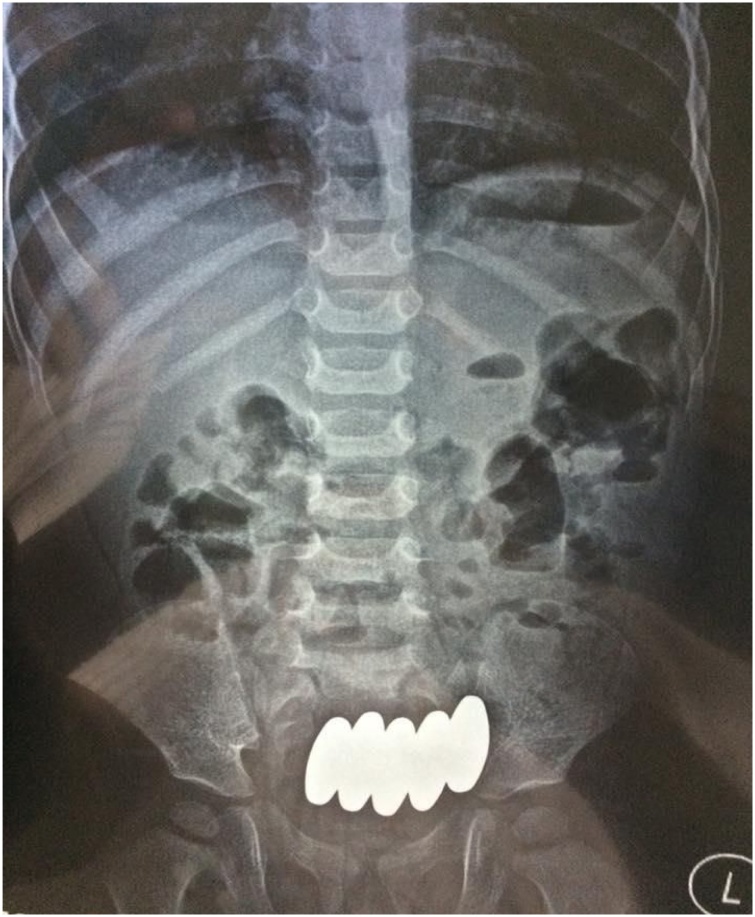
An erect abdominal X-ray showing five bullet shaped magnets in the lower abdomen with no air-fluid levels or air under the diaphragm.
